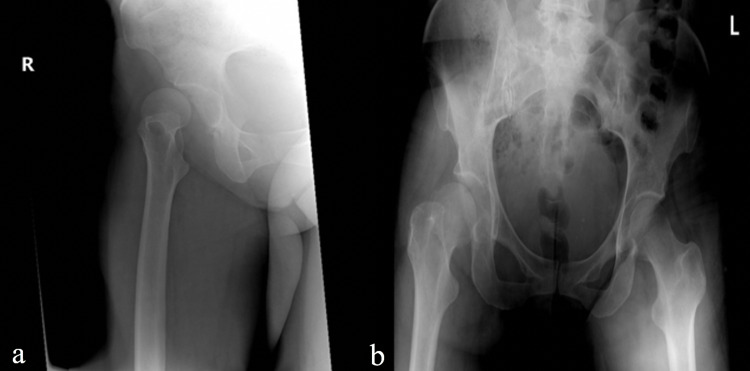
Radiographs of the right hip and pelvis showing anterior hip dislocation. (a) Anteroposterior (AP) view of the right hip. The radiograph demonstrates that the femoral head has dislocated from the acetabulum in an anterior direction. (b) AP view of the right pelvis. This radiograph also shows that the right femoral head is dislocated compared to the contralateral side.
